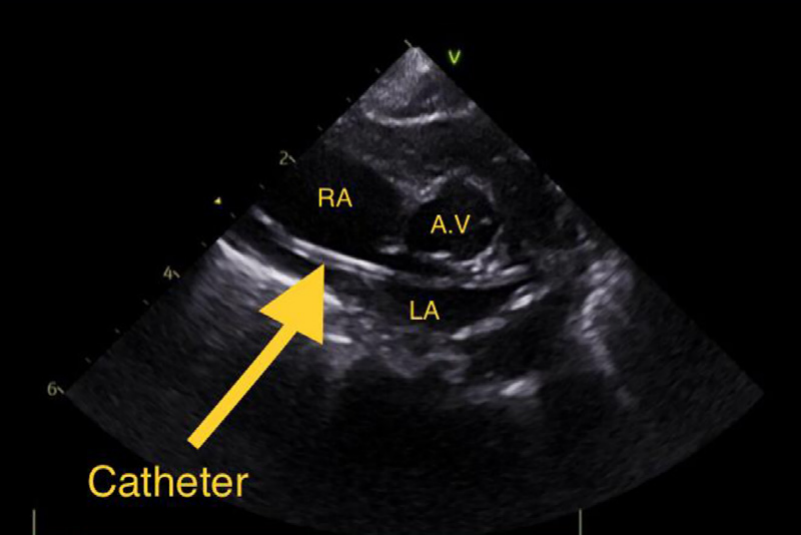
Parasternal short axis view of the transthoracic echocardiography shows the catheter (arrow) in the right atrium passing through patent foramen ovale into the left atrium. RA: Right atrium; LA: Left atrium; A. V: Aortic valve.
